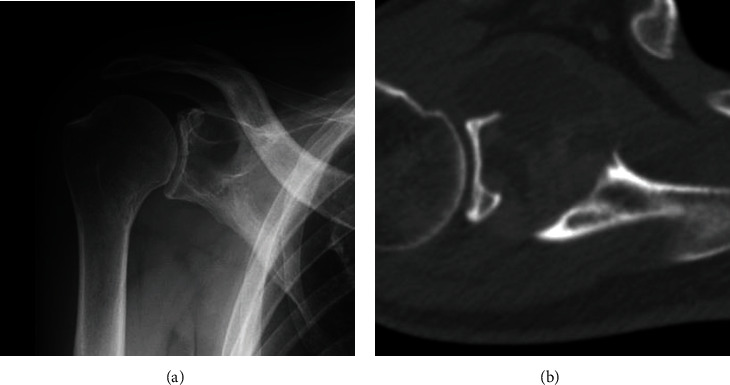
(a) Conventional radiograph of the right shoulder showing a lytic lesion involving the right scapula near the glenoid. (b) Computed tomography depicting the osteolytic lesion at the scapula with associated cortical destruction and extension into the surrounding soft tissue.
